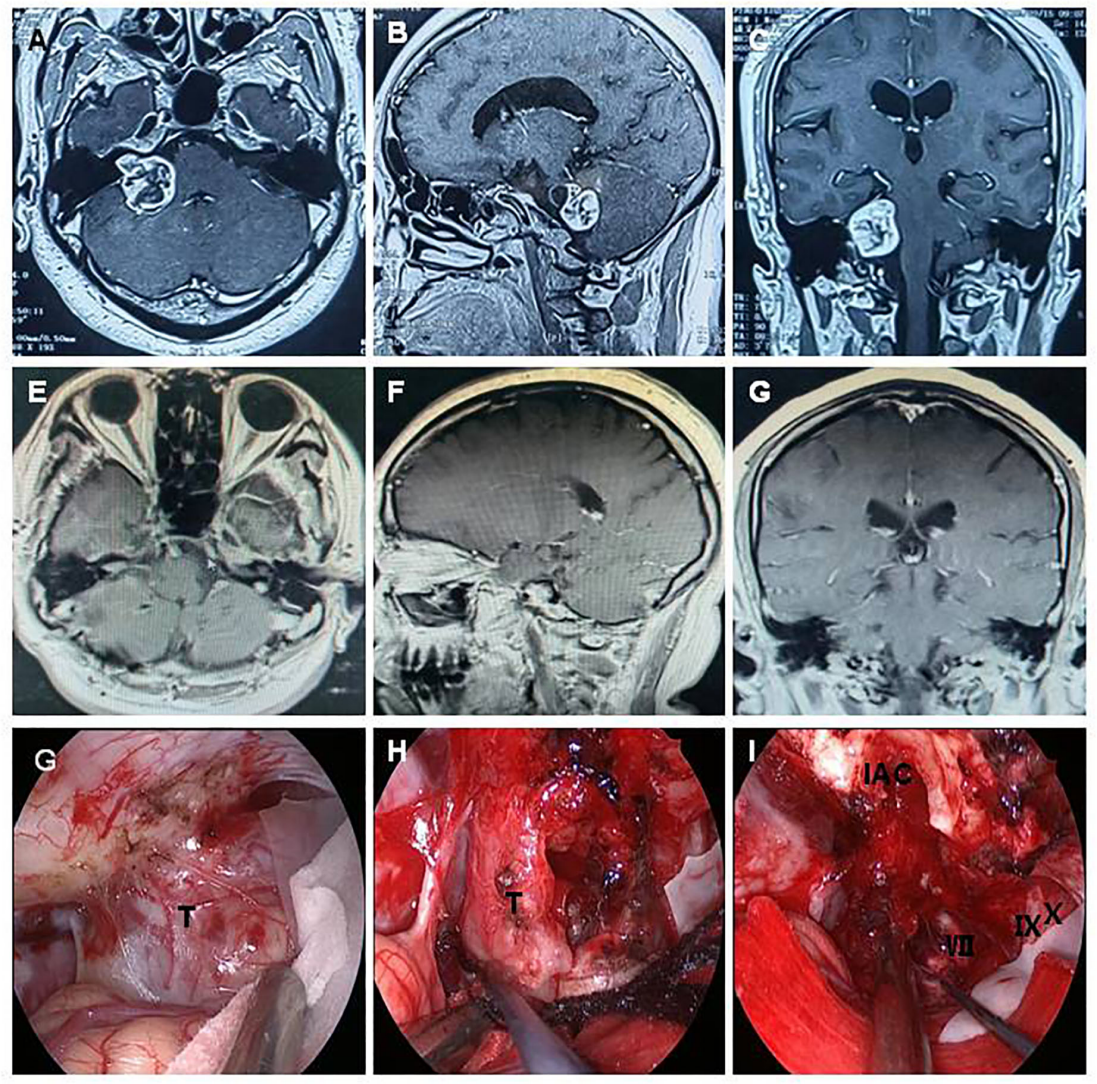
A 54-year-old woman presented with acoustic neuroma. A preoperative MRI scan demonstrated a − 3.3 cm × 2.5 cm lesion located in the CPA region, showing heterogeneous enhancement (A–C). The tumor was completely resected using a fully endoscopic retrosigmoid approach. GTR of the CPA region tumor was confirmed by MRI at 3 months postoperation (D–F). The fully neuroendoscopic surgical procedure demonstrated tumor exposure (G), tumor dissection (H), and complete tumor resection with preservation of the facial nerve (I). T, tumor; VII, facial nerve; IX, glossopharyngeal nerve; X, vagus nerve; IAC, internal acoustic canal.
